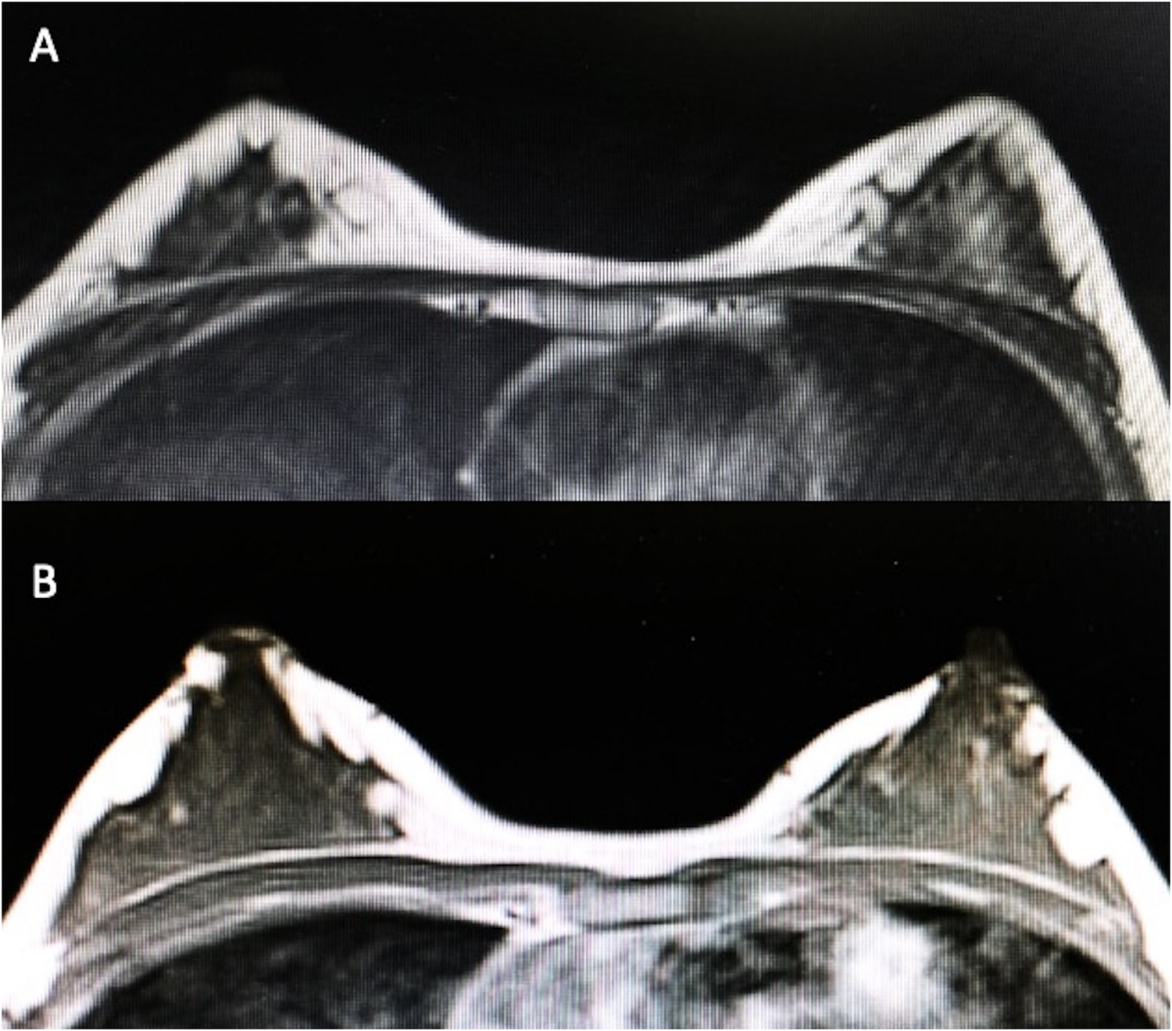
T2-weighted MRI scans of the CG patient showed in Fig. 3. A Pre-operative situation of the patient with a high degree of breast hypoplasia bilaterally. B Post-operative obtained 6 months later the second fat graft injection with volume improvement in the breasts bilaterally
